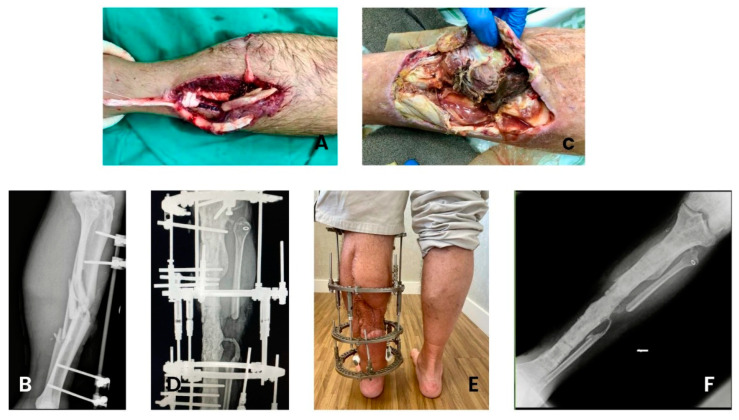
(A) Open Gustilo–Anderson IIIB fracture of the left tibia diaphysis. (B) Orthopaedic damage control with surgical cleaning, debridement, and linear external fixator. (C) Severe musculoskeletal infection with ischaemia and necrosis of fascia, muscles, and osteomyelitis. (D) Treatment of the bone defect with bioactive glass S53P4, bone lengthening, and linear external fixator. (E) Patient underwent soft tissue grafting and while remaining with circular external fixator. (F) X-ray showing osteointegration of bioactive glass S53P4 and removal of the external fixator.
